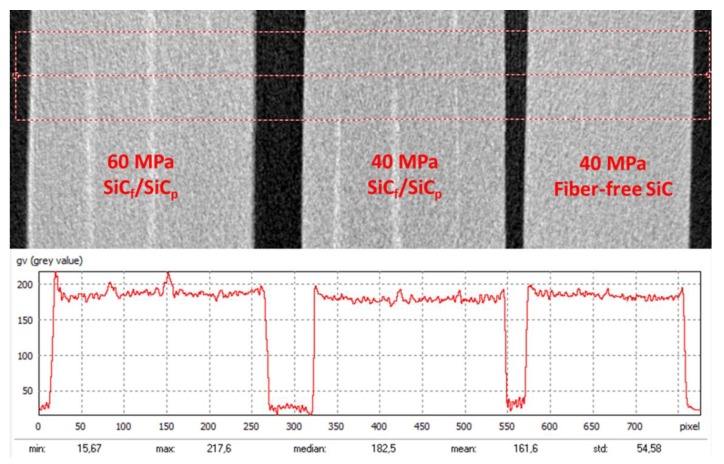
The X-ray density of the samples by the integrated profile.
